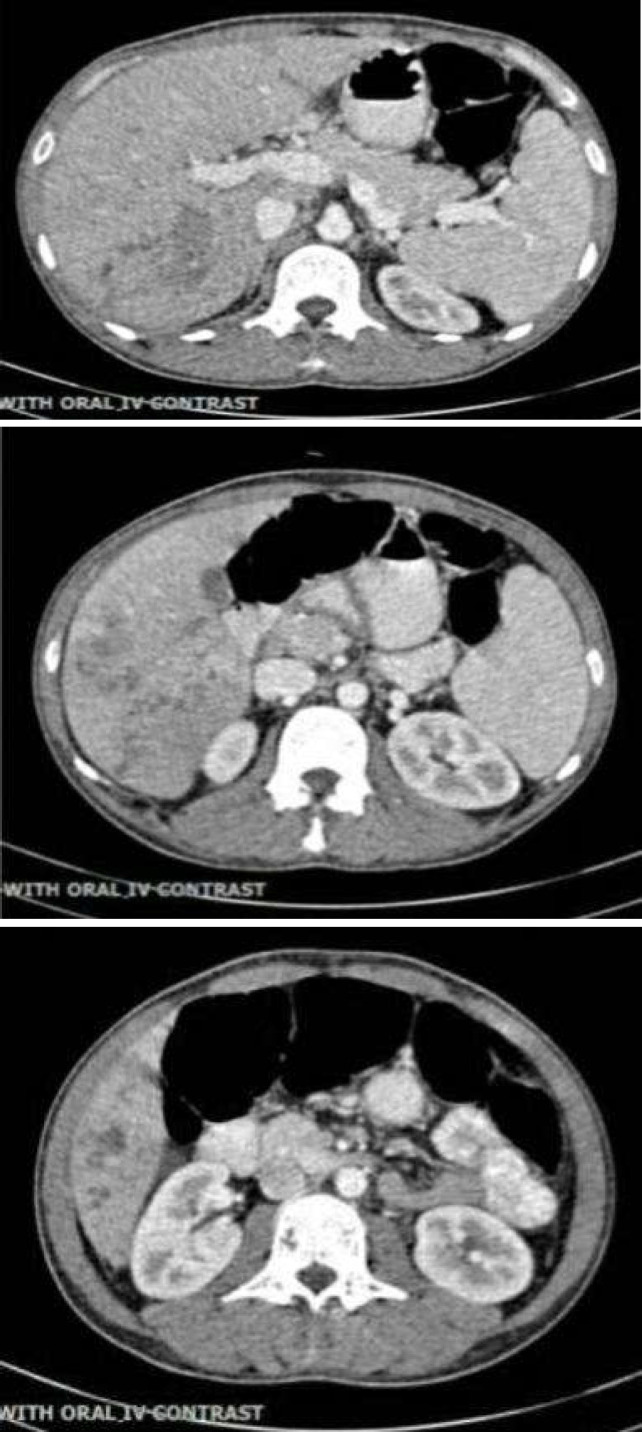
Abdominopelvic CT scan demonstrates disseminated intrahepatic duct dilation and periductal enhancement of the liver parenchyma predominantly in right liver lobe
